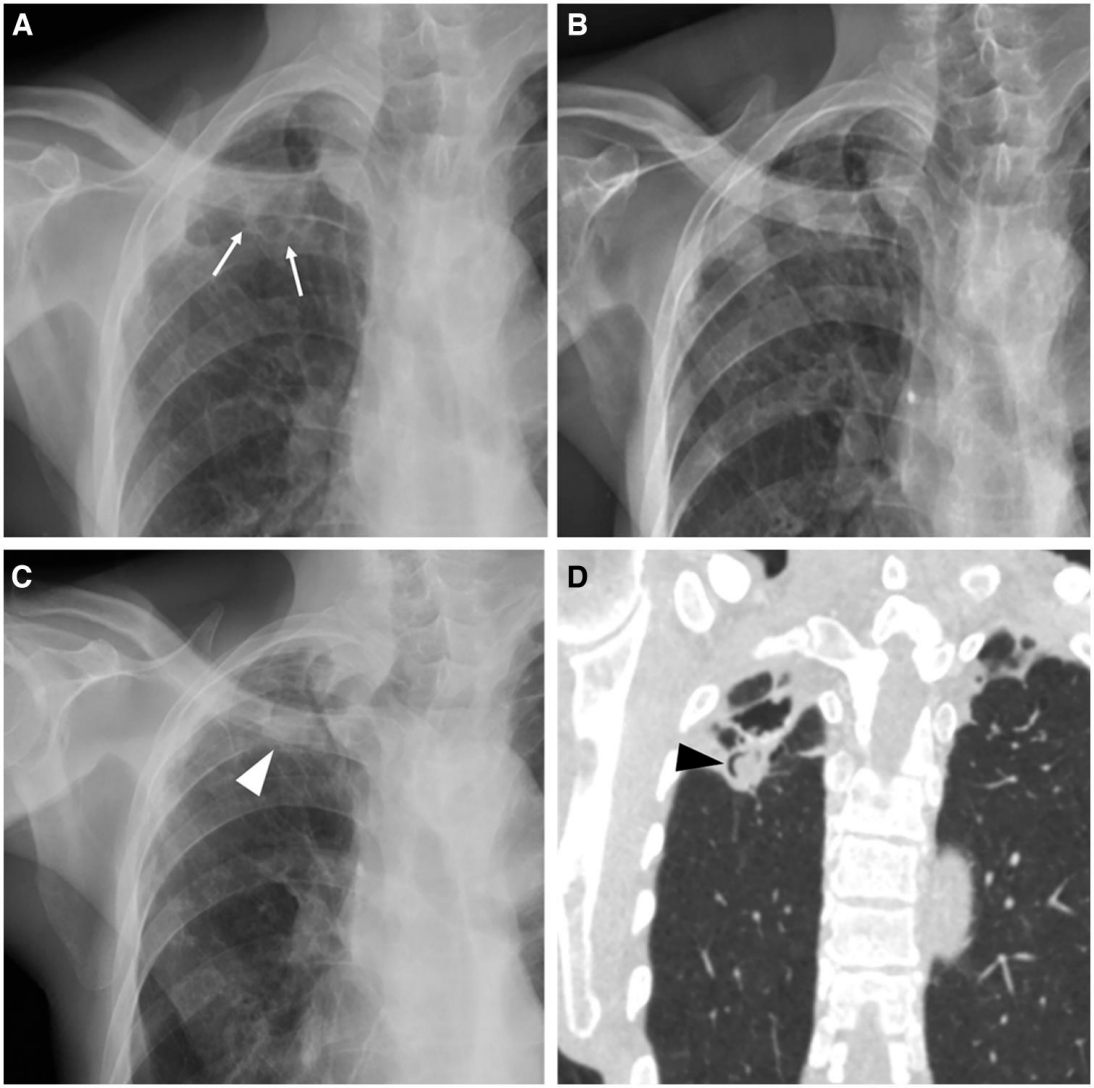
Postprimary pulmonary TB with cavitation and subsequent aspergilloma in an 88-year-old man. (A) Frontal chest radiograph shows a thick-walled cavitary lesion in the right upper lobe (white arrows). (B) Follow-up radiograph 2 years later shows healing with fibrosis. (C) Follow-up radiograph 5 years later shows development of a rounded opacity (white arrowhead). (D) Coronal CT image shows a fungal ball in the parenchymal cavity with an air crescent sign (black arrowhead). There is adjacent upper lobe fibrosis.
